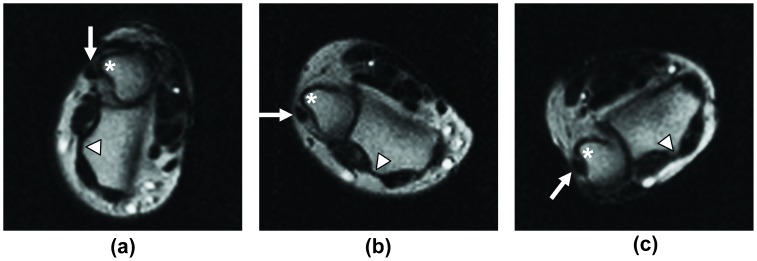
Extensor carpi ulnaris (ECU) tendon translation during wrist rotation. The relationship of the ECU tendon (arrow) to its groove as the forearm is rotated from (a) pronation, through (b) neutral to (c) supination – on axial images of the DRUJ using the active-MRI scan. In this volunteer, the ECU tendon was located within its groove in pronation, while in the neutral position, the tendon is subluxated eccentrically at the margin of the ulnar groove. In supination, the tendon is dislocated. Also visualized is the trajectory of the ulnar styloid process (white star) during the supination/pronation maneuver. Lister's tubercle (white triangle) at the dorsal aspect of the radius is shown as an anatomical reference point.
